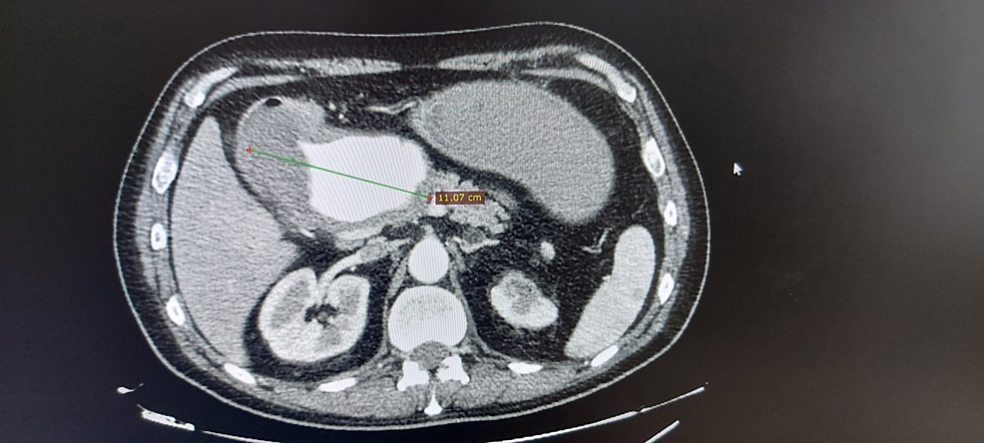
Contrast-enhanced CT axial view of the giant hepatic aneurysm. CT: computed tomography
